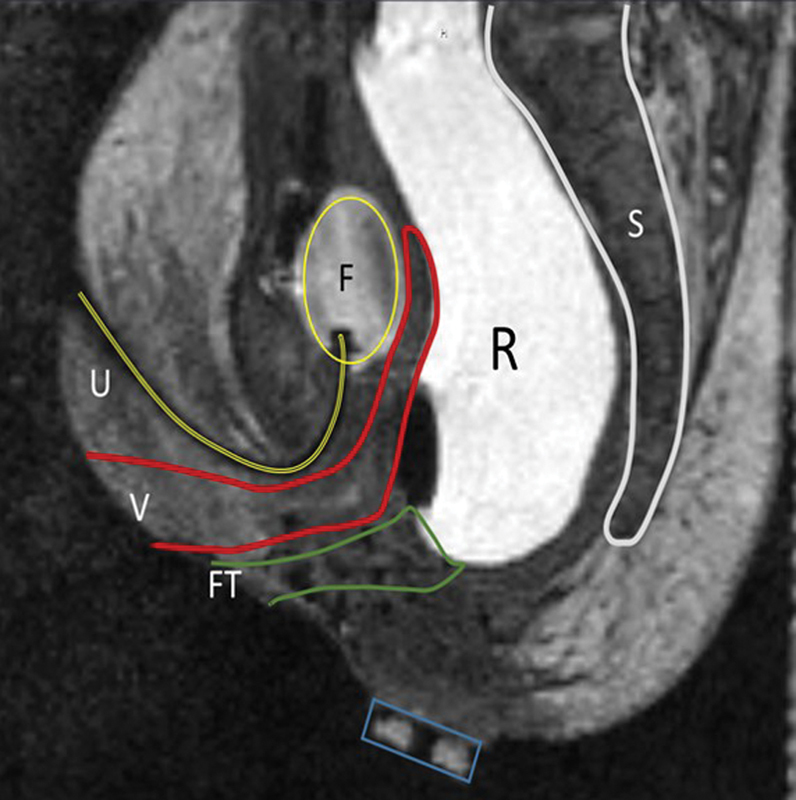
Sagittal magnetic resonance image (MRI) of the patient with failed primary posterior sagittal anorectoplasty for vestibular fistula. F, Foley; FT, previous fistula tract; R, rectum; S, sacrum; U, urethra; V, vagina.
